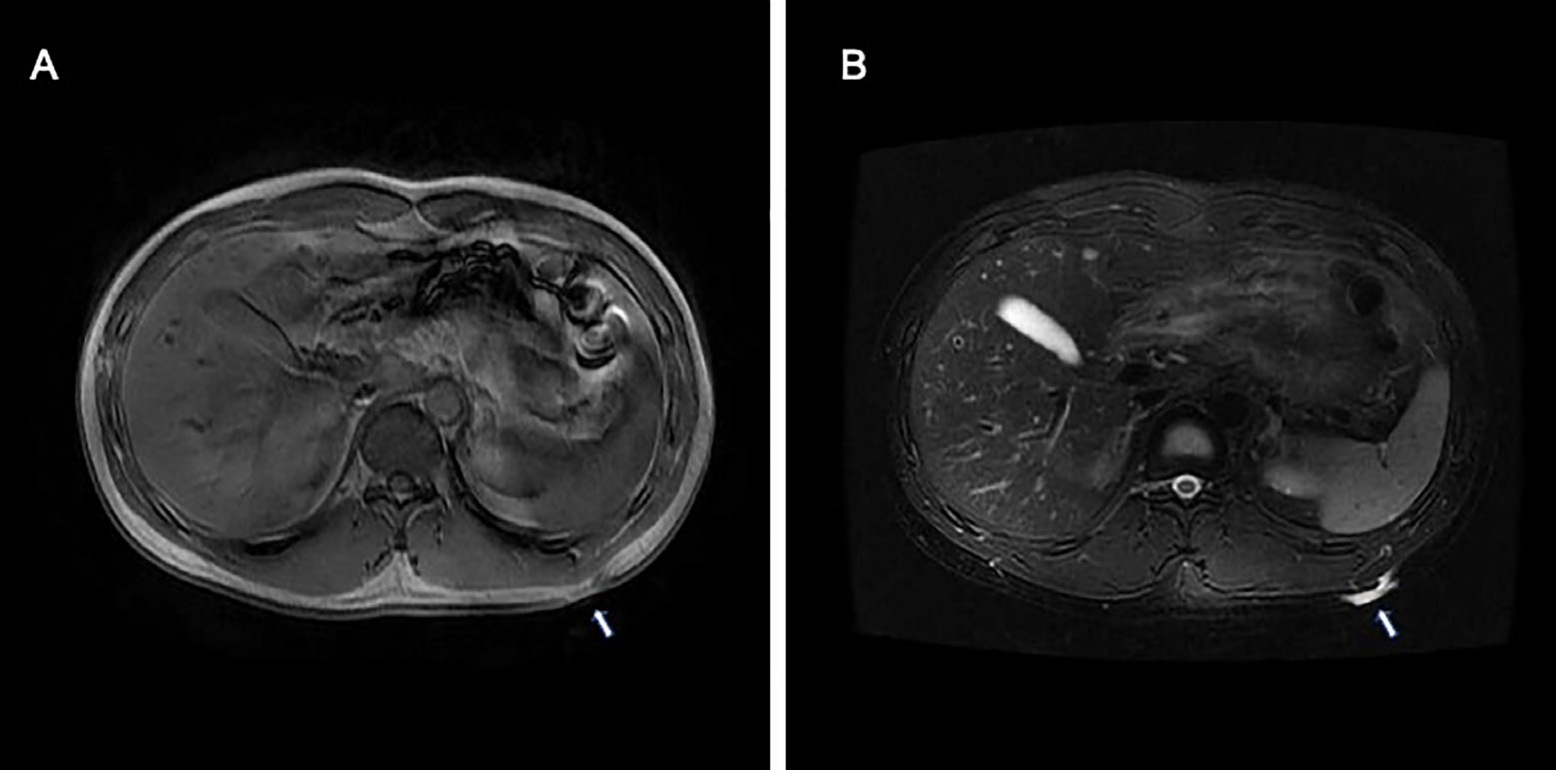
Magnetic resonance imaging of atrophic pigmented DFSP showed a well-circumscribed plaque with adjacent tissue thinning in the left region of the trunk. (A) T1-weighted images revealed a slightly higher non-uniform signal, (B) while T2-weighted images showed a high signal.
